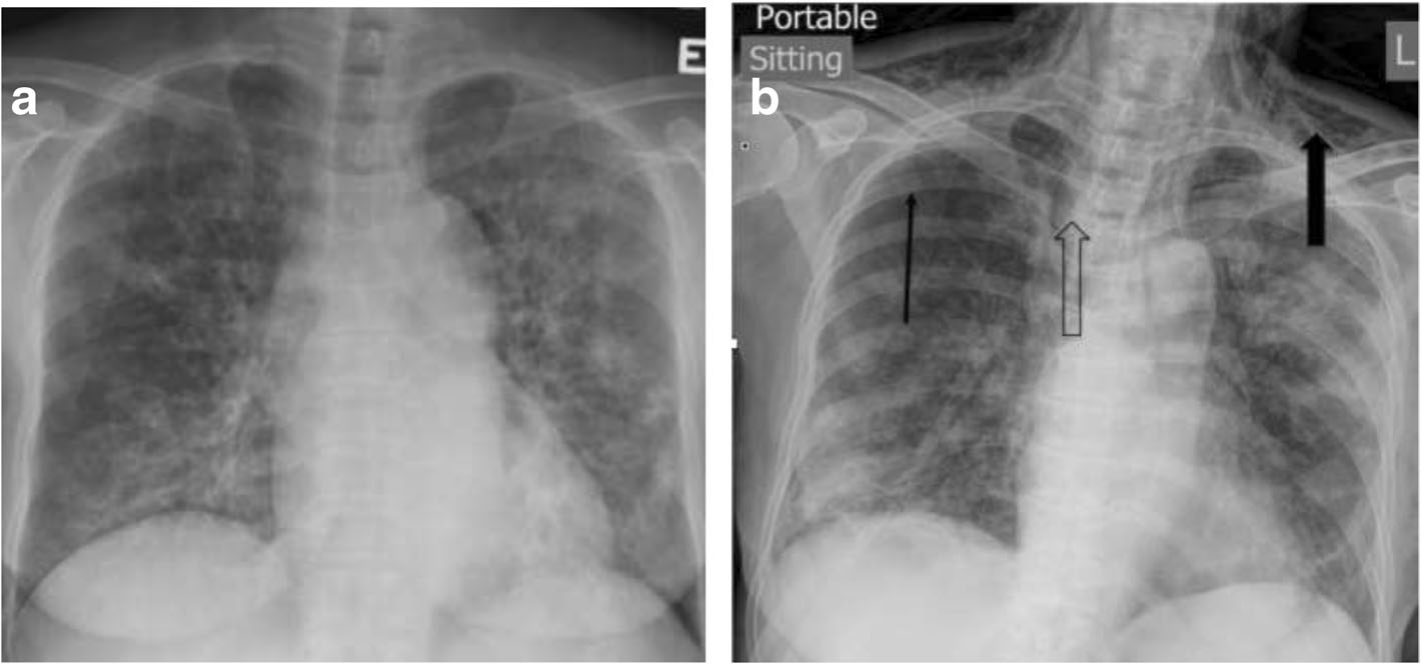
a Chest X-ray on admission showing bilateral peri-hilar interstitial infiltrates. b Chest X-ray showing right pneumothorax (thin arrow), subcutaneous emphysema (solid arrow) and pneumomediastinum (hollow arrow)
